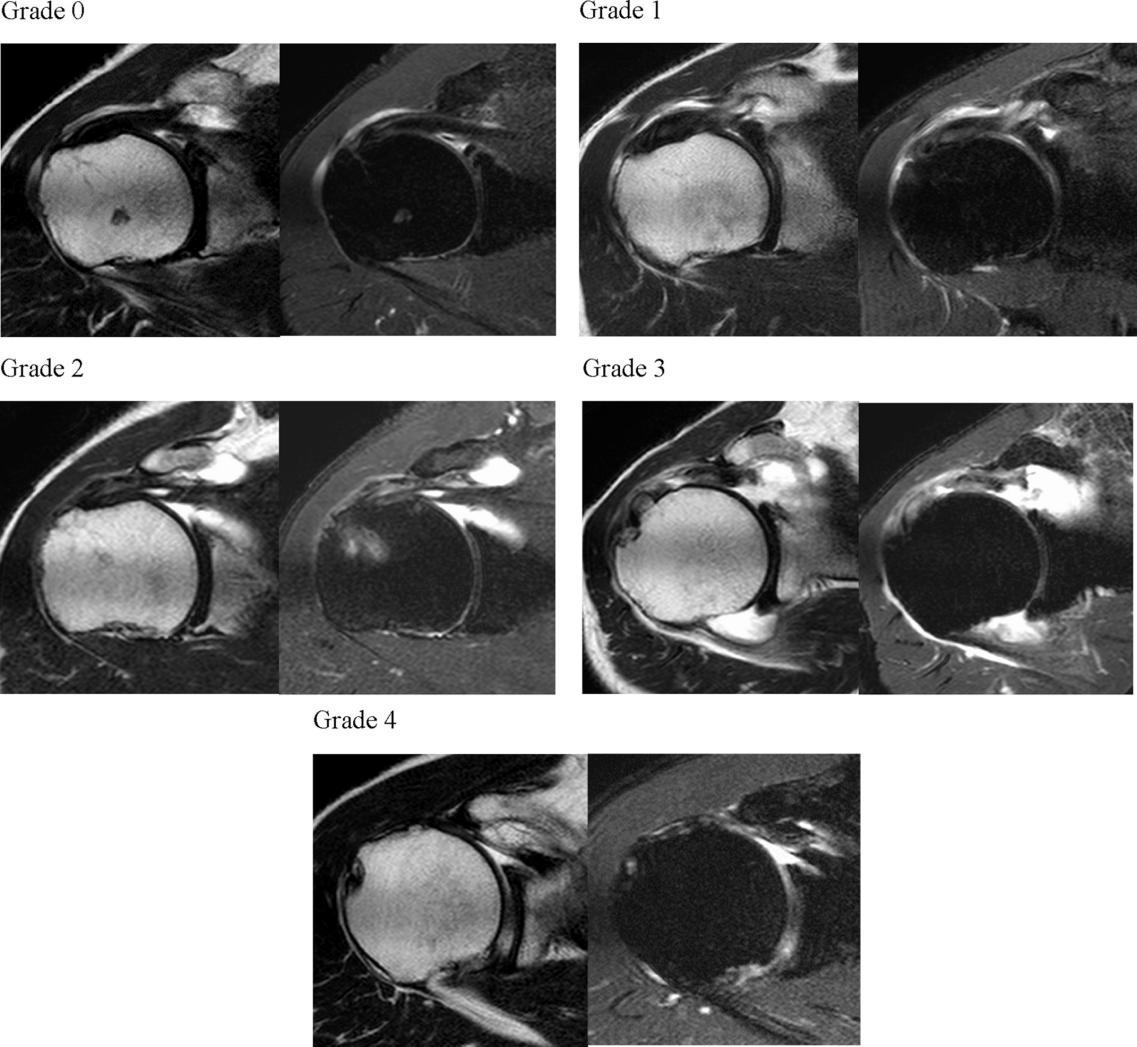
rMRI images of the classification of the anterosuperior aspect STT. Grade 0, intact; Grade 1, slight thinning of the SSC; Grade 2, considerably thinning of SSC; Grade 3, complete tear; Grade 4, coracoid process contact and anterior potion of humeral head. The *Left* images are T2-weighted, and the *Right* images are T2FS. *rMRI*, radial-sequence MRI; *STT*, subscapularis tendon tear; *SSC*, subscapularis tendon.
